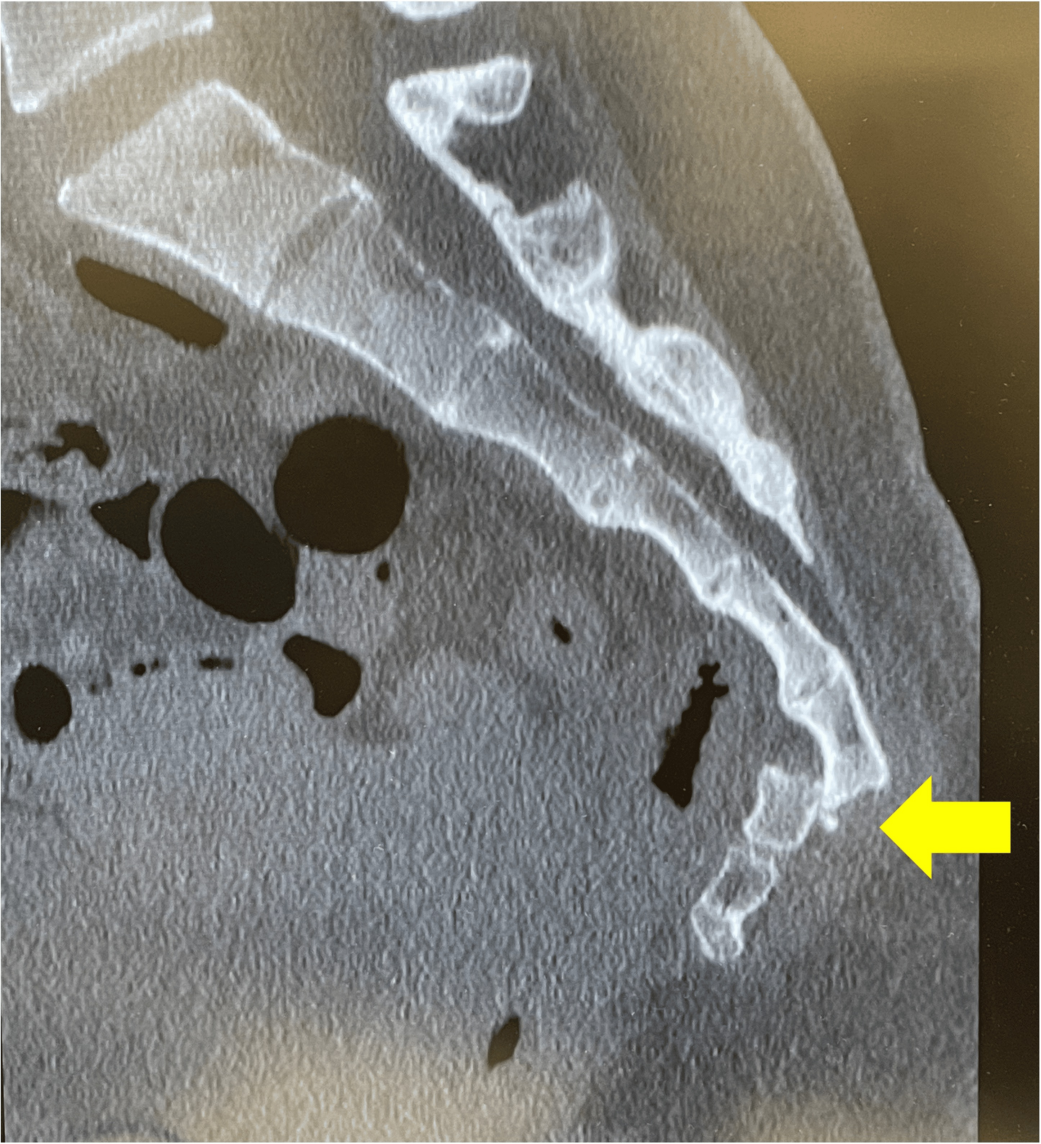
Imaging at the time of injury. Sagittal computed tomography (CT) slice showing a small bone fragment at the dislocation site (arrow).
